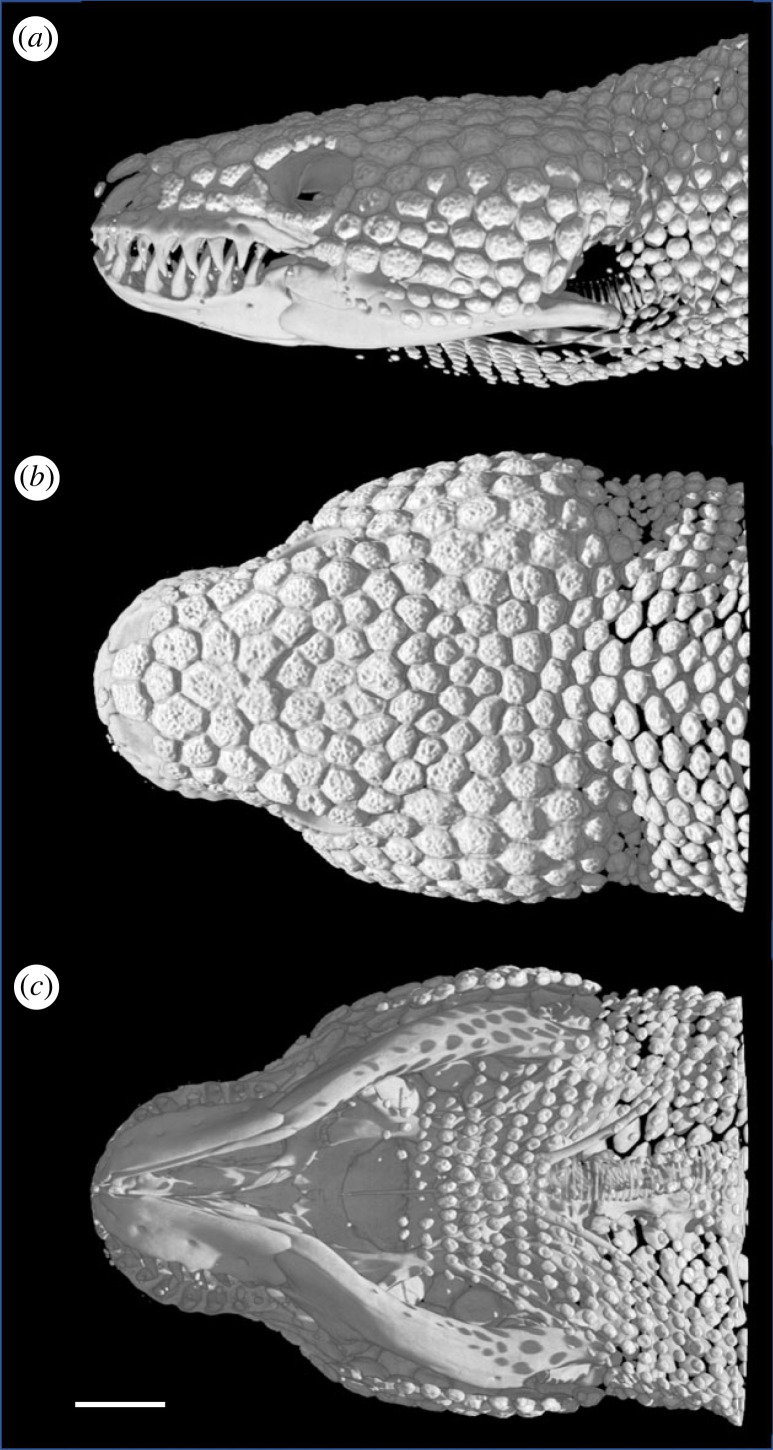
Osteoderms of the Gila monster. CT scans of the (*a*) lateral, (*b*) dorsal and (*c*) ventral aspects of the head of an adult *H. suspectum*. The top and sides of the head are well protected by large, closely spaced osteoderms. Osteoderms in the posterior gular region are relatively few and small, and the lower jaw completely lacks them. Note the formidable teeth of these lizards (*a*). Scale bar = 1 cm. Images courtesy of DigiMorph.com.
